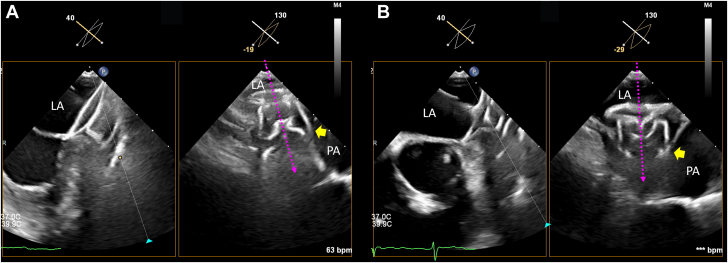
TEE Assessment for Pulmonary Artery (A) Initial deployment oriented the lobe tip toward the 5 o'clock position on a 130° TEE view (magenta dotted arrow). The LAA wall pressed against the pulmonary artery at the level of the distal lobe anchors (yellow arrow), increasing the contact surface between the anchors and the artery and raising concern for DR-PAI (Video 5). (B) After recapturing the device, the second deployment rotated the tip toward the 6 o'clock position at 130° (magenta dotted arrow), thereby reducing pulmonary artery contact and mitigating impingement risk (yellow arrow) (Video 6). DR-PAI = device-related pulmonary artery impingement; LA = left atrium; LAA = left atrial appendage; PA = pulmonary artery; TEE = transesophageal echocardiograph.
